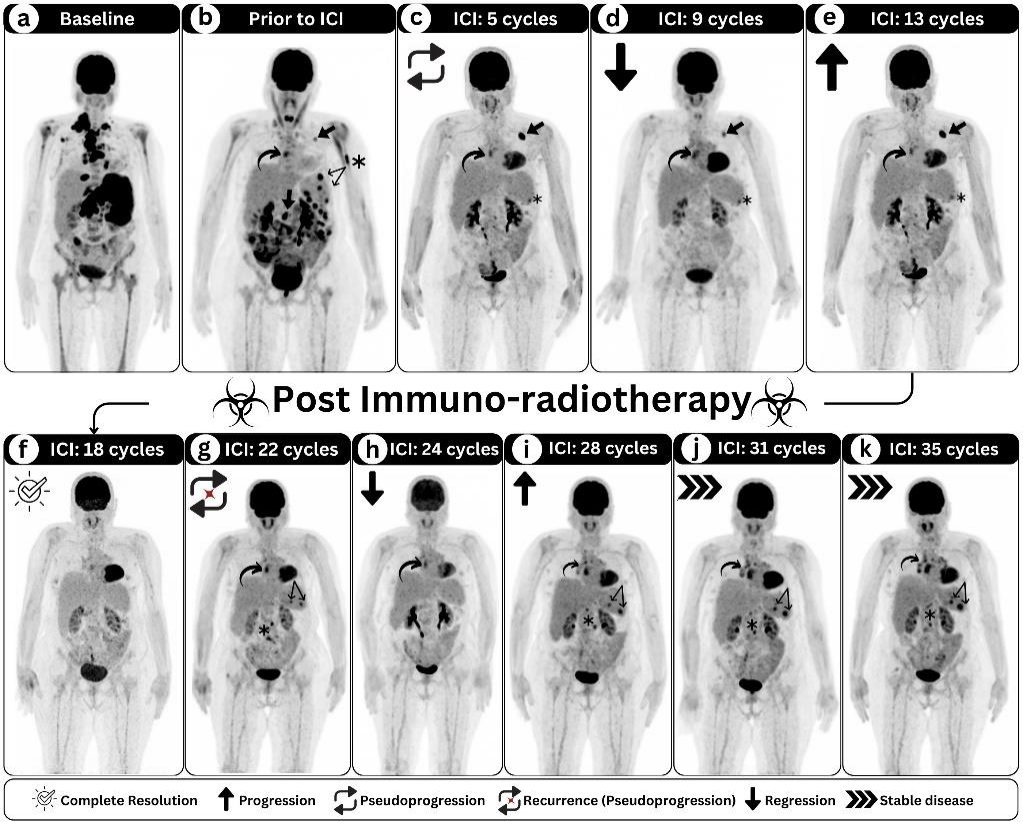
(a- k) Serial maximum intensity projection images of ^18^F-FDG PET/CT offered prior to immunotherapy initiation up until administration of 35 cycles of pembrolizumab
